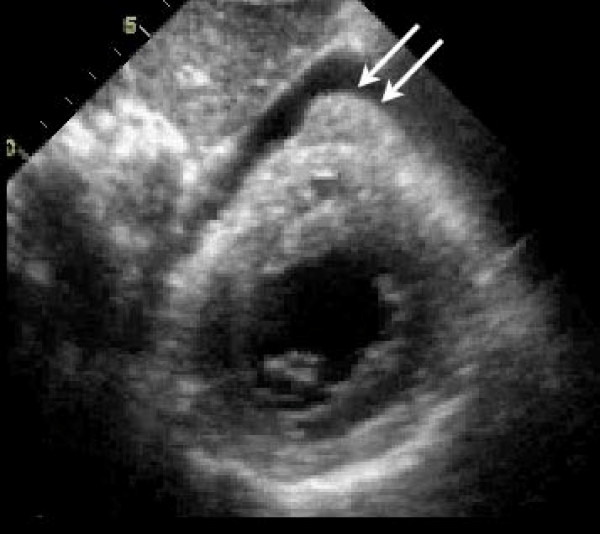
A transthoracic echocardiographic short axis view from subxiphoid view representing echodense material attached to the right ventricular wall, presumed to be thrombus (arrows).
